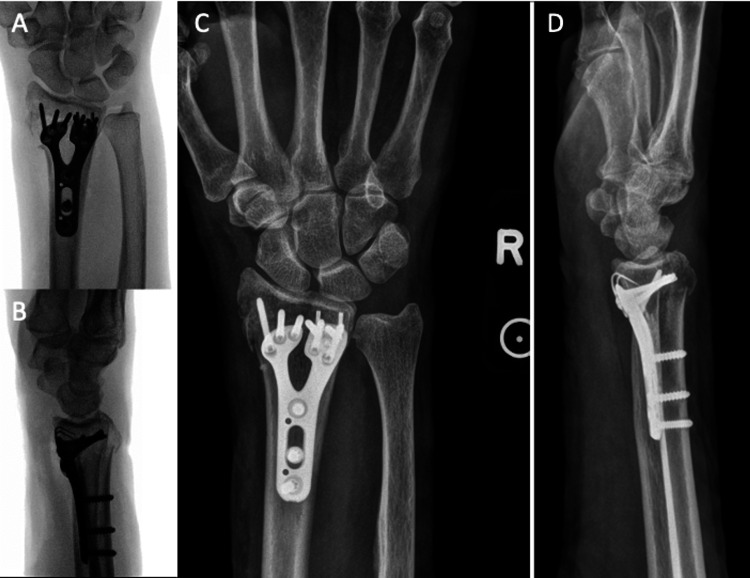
Intraoperative and postoperative films. A-B) intraoperative fluoroscopy. C-D) two-week postoperative anteroposterior and lateral X-rays views of the right wrist: volar plate and hook plate appearing intact, with the restoration of the articular surface, radial height, and radial tilt.
